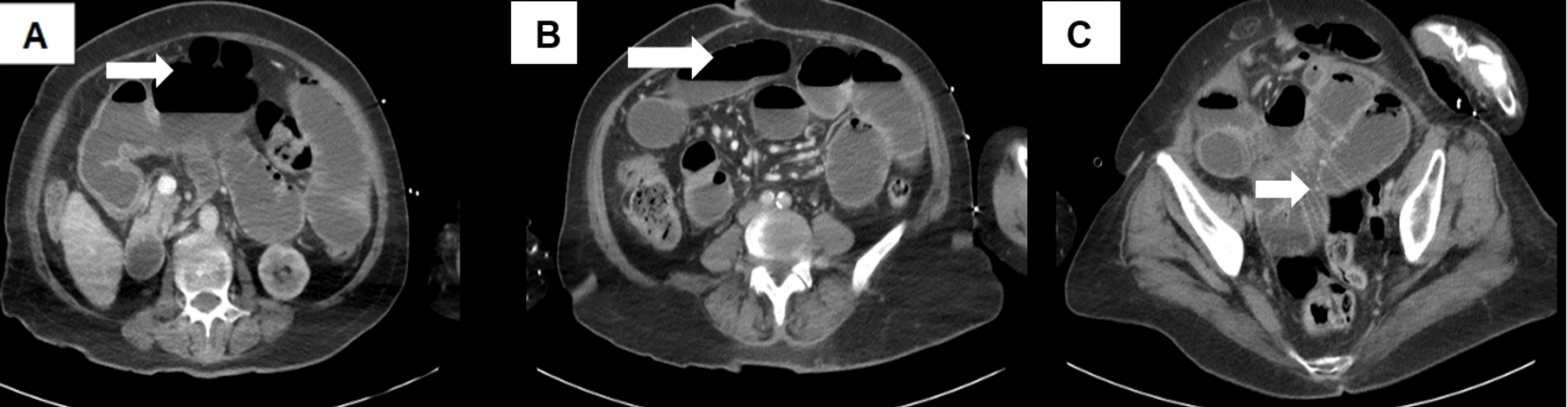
CT scan on admission (A, B) demonstrate dilated small bowel with an air-fluid level. (C) demonstrates adhesions within the small bowel. CT: Computed tomography
